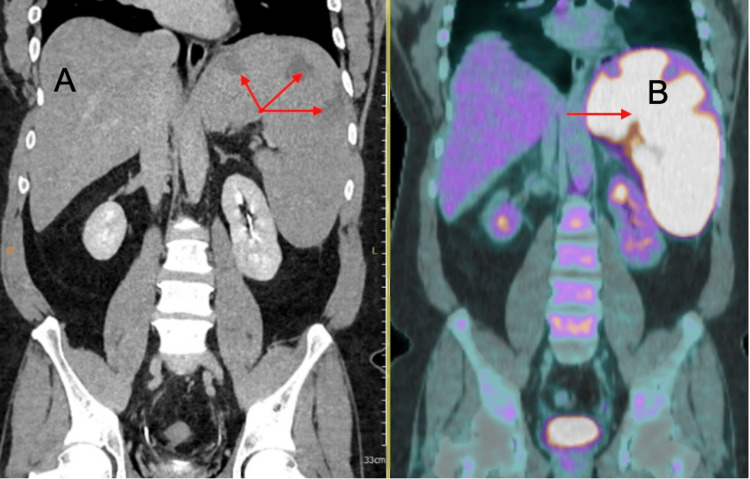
Coronal CT and PET scan A (left): Coronal CT image showing marked splenomegaly and areas of low attenuation consistent with splenic infarcts (red arrows). B (right): A fludeoxyglucose (FDG) PET/CT scan demonstrating increased FDG uptake in the spleen (red arrow), suggestive of lymphomatous involvement.
